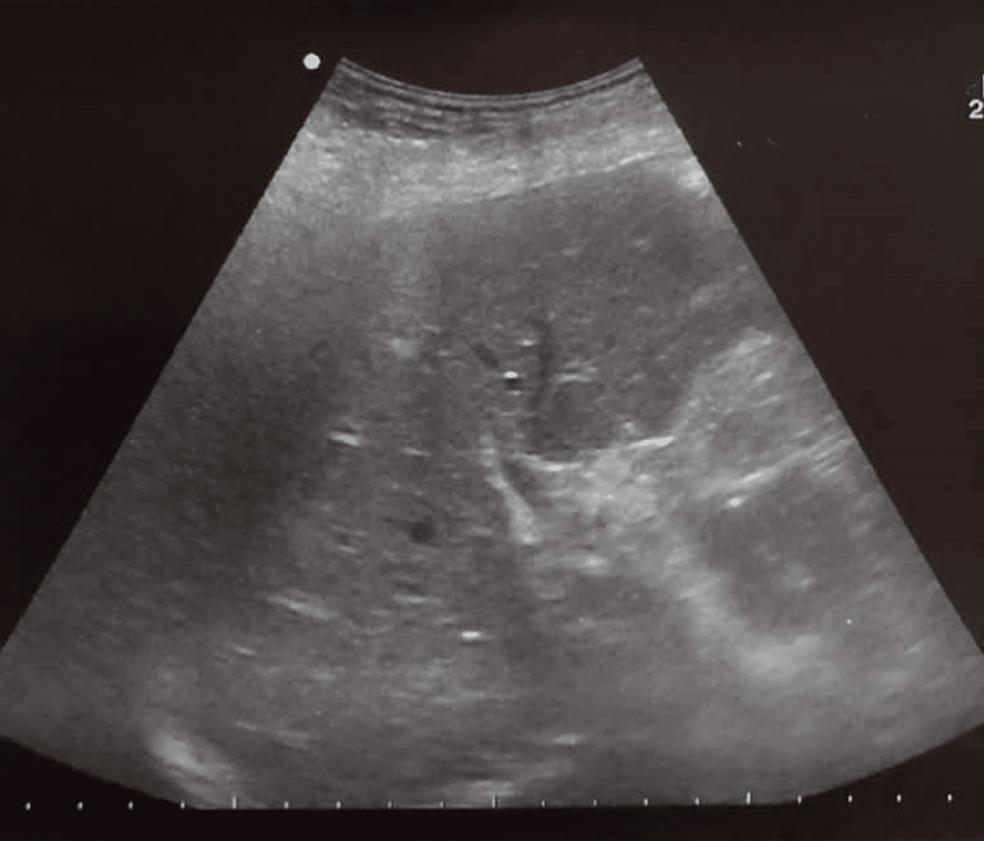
Follow-up ultrasound examination showing complete remission of the hepatic portal venous gas.
